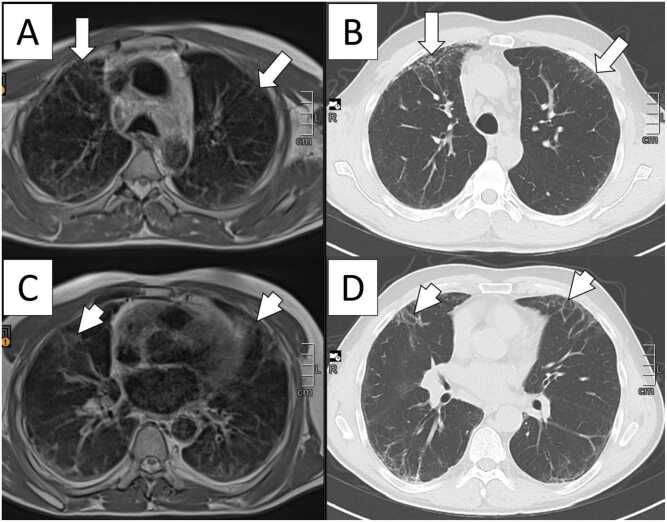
Clinical Case #2: 52-year-old male patient with systemic sclerosis-related interstitial lung disease with predominantly reticular pattern who underwent (A, C) low-field MRI of the lung with a proton density weighted sequence and (B, D) HRCT of the chest on the same day. The depicted scoring levels are level 2 (carina, A and B) and level 3 (pulmonary venous confluence, C and D). Overall disease extent was estimated almost equal (MRI, median 36 %; HRCT, median 33 %). The extent of ground-glass opacity was overestimated on MRI compared to HRCT (MRI, median 11.6 %; HRCT, median 6.0 %), whereas the extent of reticulation was underestimated on MRI (MRI, median 21.4 %; HRCT, median 27.0 %). At level 2 (A, B), reticulation in both anterior upper lobes can be depicted on HRCT as well as on MRI; however, it is less extensive on MRI (long arrows). At level 3 (C, D), reticulation on MRI becomes less clearly depictable and rather appears as a ground-glass opacity-like pattern (short arrows).
